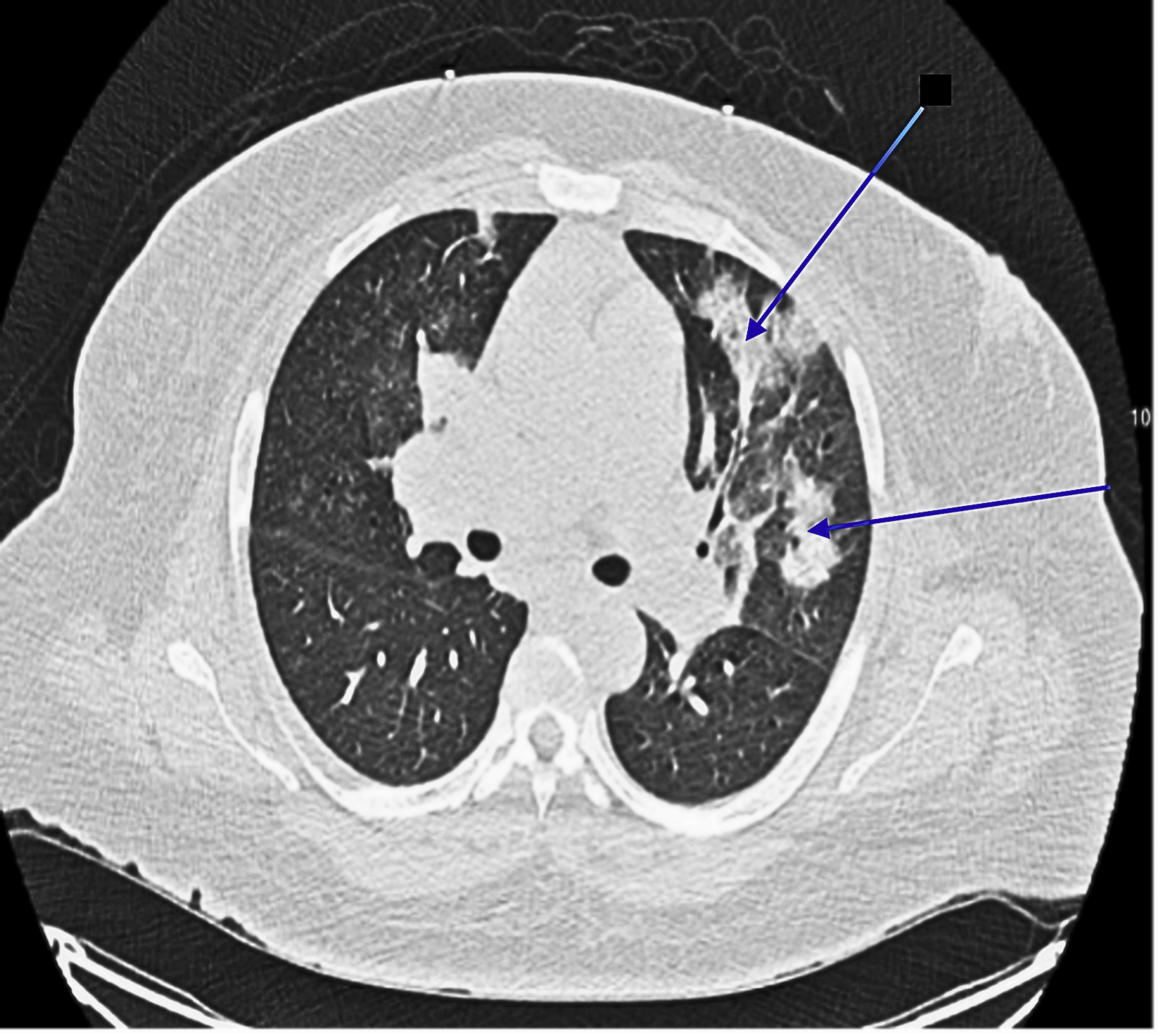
CT scan showing left-sided opacifications (marked by blue arrows in the axial view)
